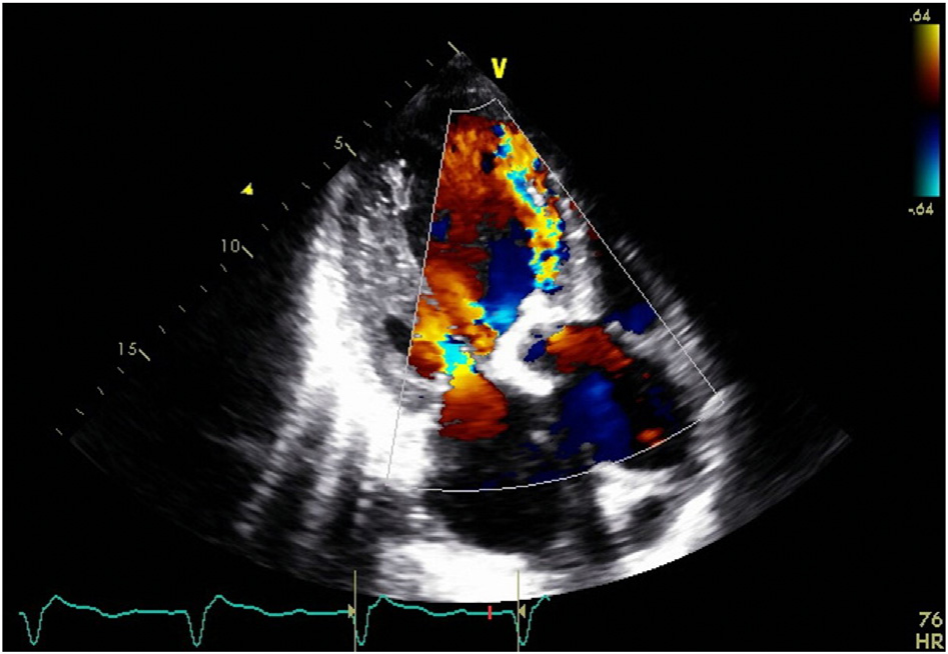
Transthoracic 3-chamber view with color Doppler across the bioprosthetic aortic valve during end diastole. Eccentric regurgitation jet as well as diastolic rocking of the valve in the left ventricle compared to end-systolic valve position.
